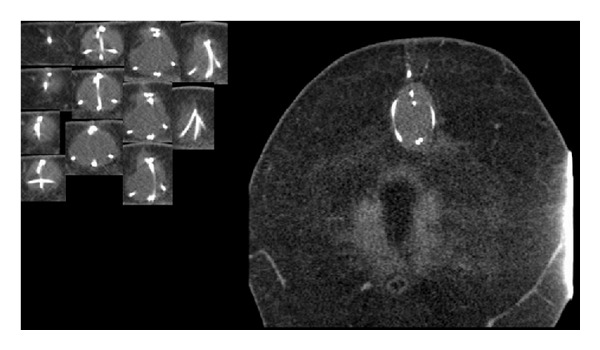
CT images from first VP shunt extrusion.
